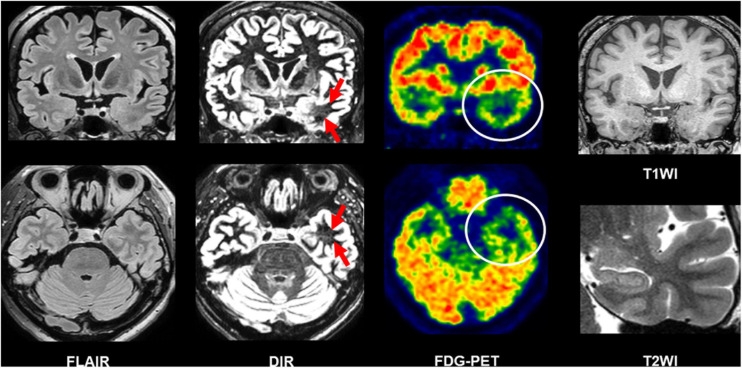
A case of conventionally MRI-negative PET-positive temporal lobe epilepsy (TLE). While it was difficult to detect abnormalities on FLAIR, double inversion recovery (DIR) visualized hyperintensity within the anterior temporal white matter of the focus side. T1-weighted images (T1WI) and T2WI were also intact including the hippocampus.
